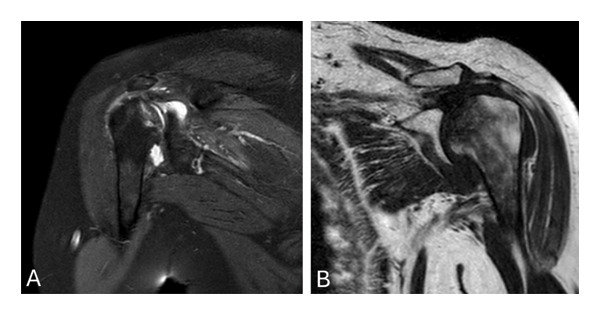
Shoulder MRI showing osteonecrosis of both the right (A) and left (B) heads of the humerus in the coronal view.
